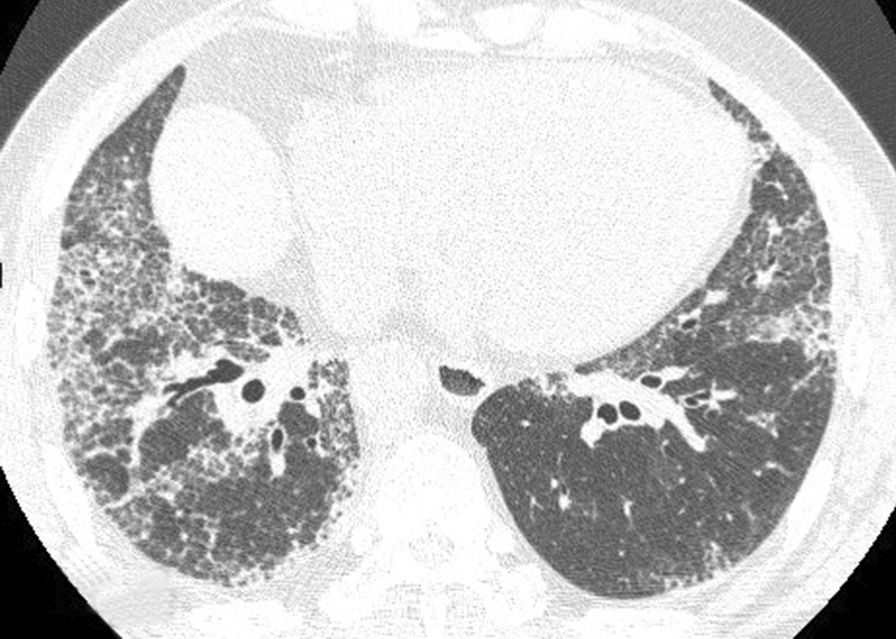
The CT of a male ILD patient in his mid 50s, who was anti-PL-7 positive, showing ground glass opacity, reticulation and traction bronchiectasis
